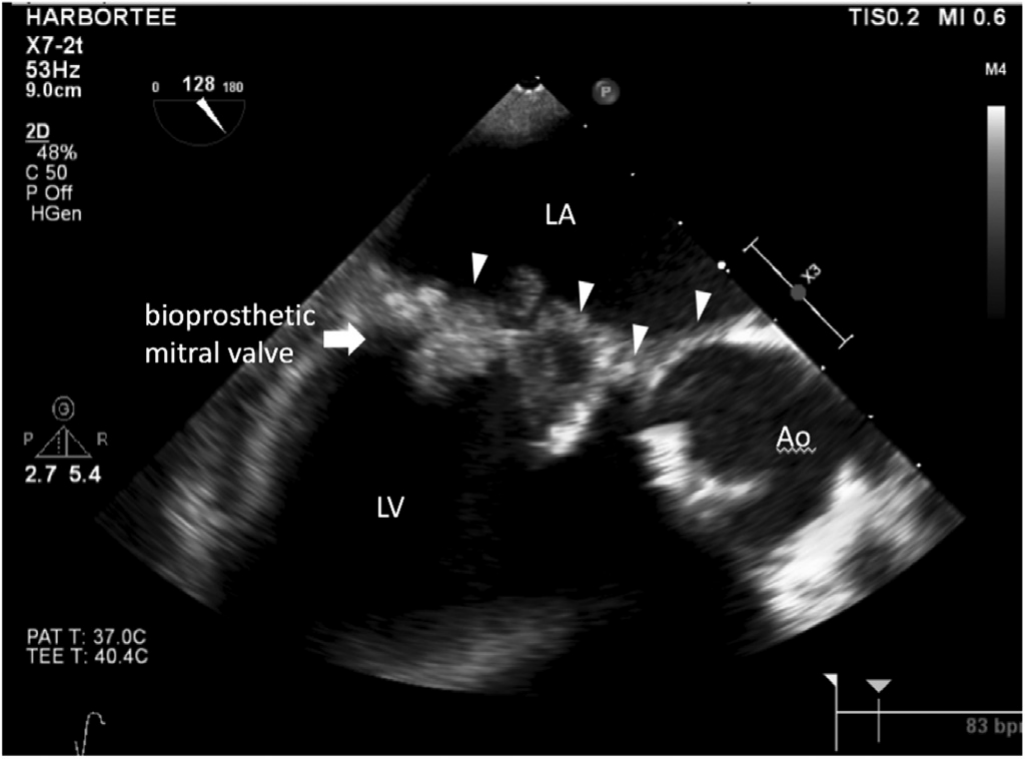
Transesophageal echocardiogram, mid-esophageal view. Shows 33 mm bioprosthetic mitral valve with extensive large, bulky vegetations encasing the leaflets. There is valve dehiscence and extension to the aortomitral curtain and aortic root (arrows). Mild.
